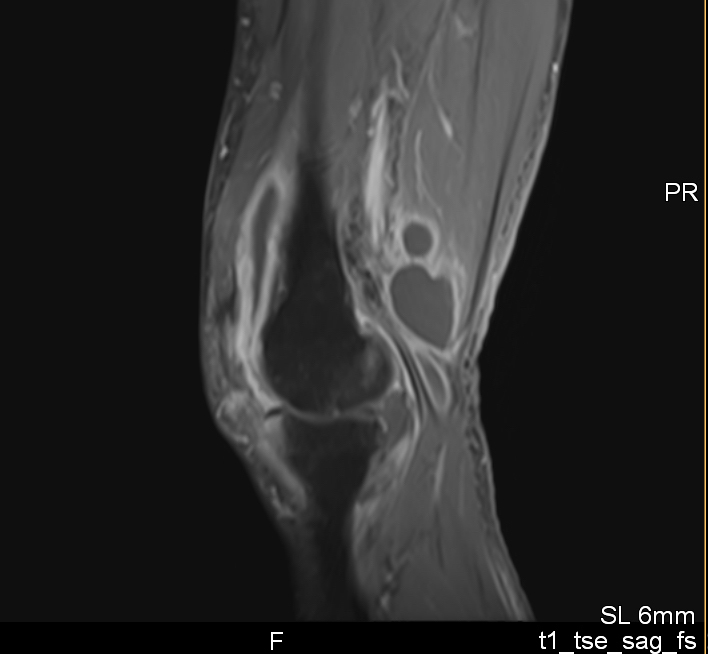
Sagittal MRI slice (T1 fat suppression) of the left knee showed massive synovitis where the thorn fragment was located.
